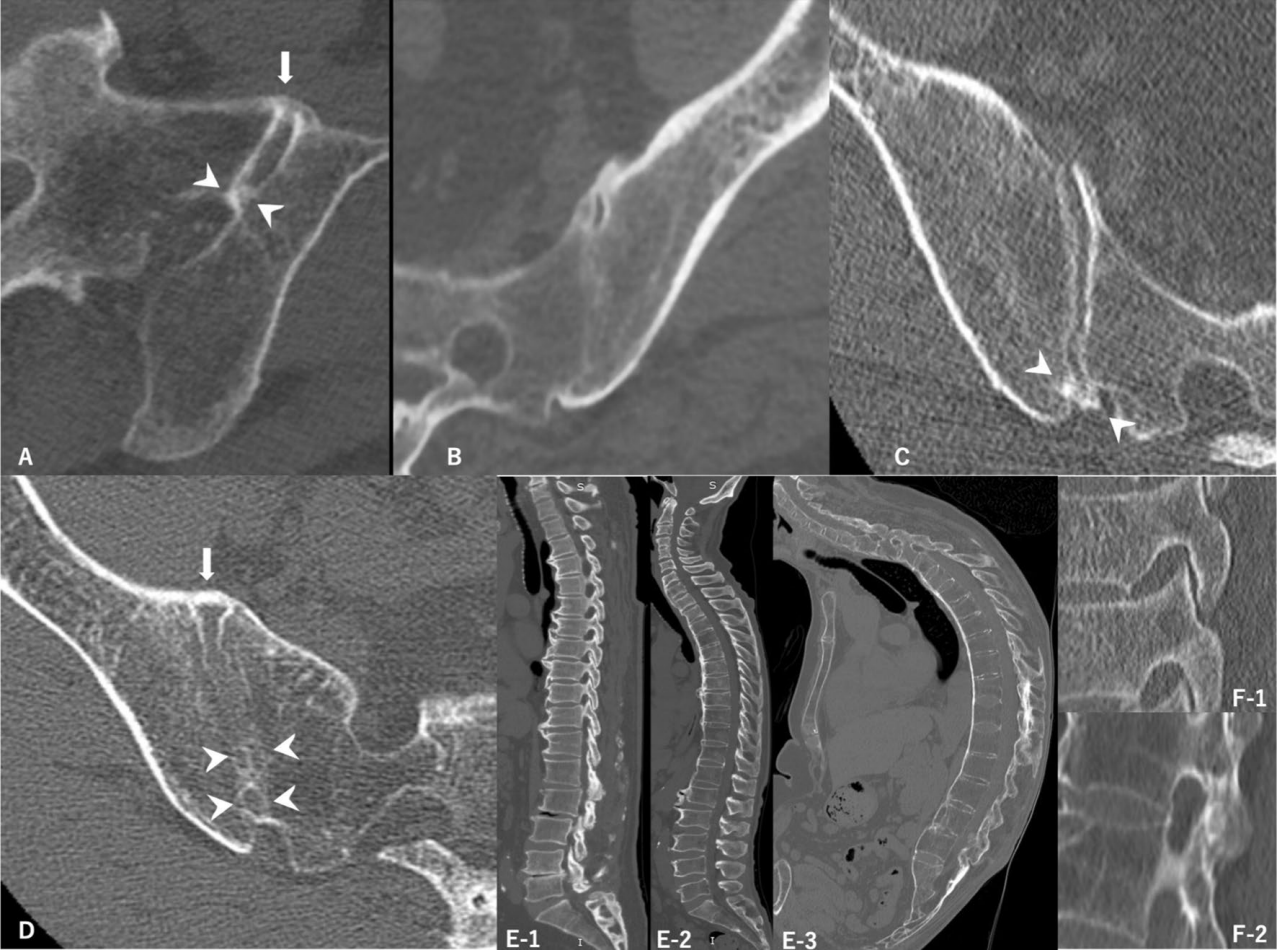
CT images of the spine and SIJ. (A) Axial CT image of SIJ shows anterior bony bridging (arrow) and partial fusion of joint (arrowhead). (B) Axial CT image of SIJ shows complete joint fusion. (C) Axial CT image of SIJ shows posterior bony bridging (arrowhead). (D) Axial CT image of SIJ shows anterior bony bridging (arrow) and entheseal bony bridging (arrowhead). (E-1) Sagittal CT image of the whole spine shows 70–100% candle-wax-type. (E-2) Sagittal CT image of the whole spine shows 30–70% candle-wax-type. (E-3) Sagittal CT image of the whole spine shows 0–30% candle-wax-type. (F-1) Sagittal CT image of the facet shows no fusion. (F-2) Sagittal CT image of the facet shows fusion.
